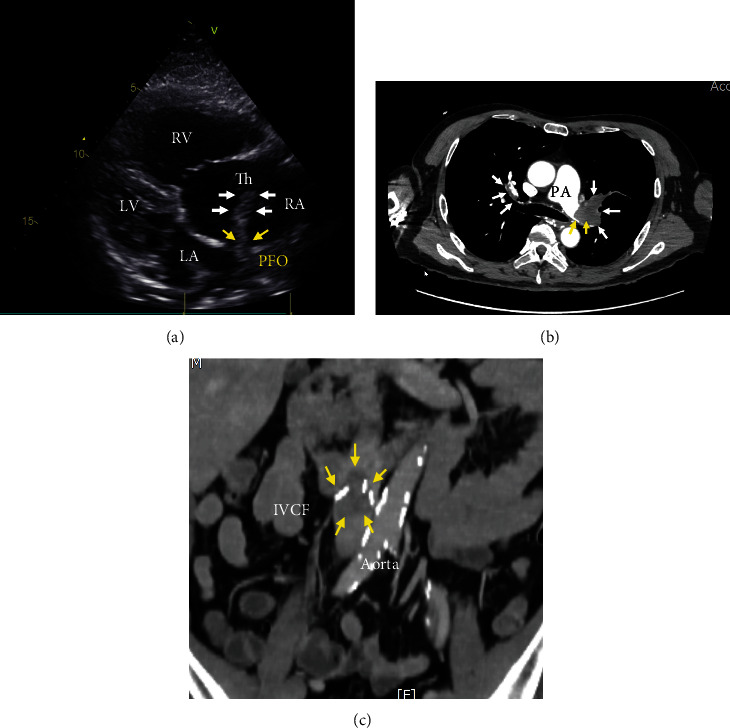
Preoperative TTE and CT findings. TTE showed dilatation of the right heart system and a newly mobile thrombus (white arrows) in the right atrium with protrusion into the left atrium through the PFO (yellow arrows (a)). CT showed extended thrombosis formation of the left main PA (yellow arrows) in addition to preexisting bilateral PE (white arrows (b)) and occluded IVCF by trapped venous thrombus (c). Yellow arrows indicated the trapped thrombus by IVCF. The thrombus was located at the proximal site of the IVCF. TTE: transthoracic echocardiography, CT: computed tomography, PA: pulmonary artery, PE: pulmonary embolism, IVCF: inferior vena cava filter, RA: right atrium, RV: right ventricle, LA: left atrium, LV: left ventricle, PFO: patent foramen ovale, Th: thrombus.
